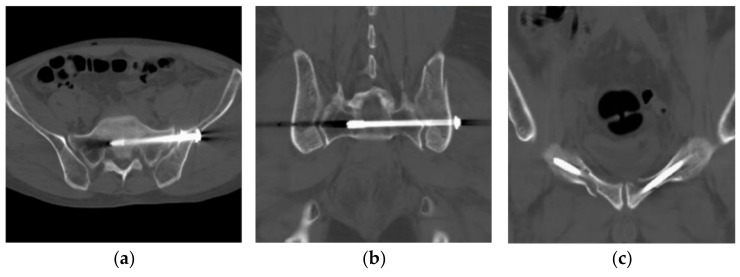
Post-operative CT scan to identify the reduction quality: (a) axial plane at the SI joint; (b) coronal plane at the SI joint view; (c) coronal plane at the anterior fracture site.
